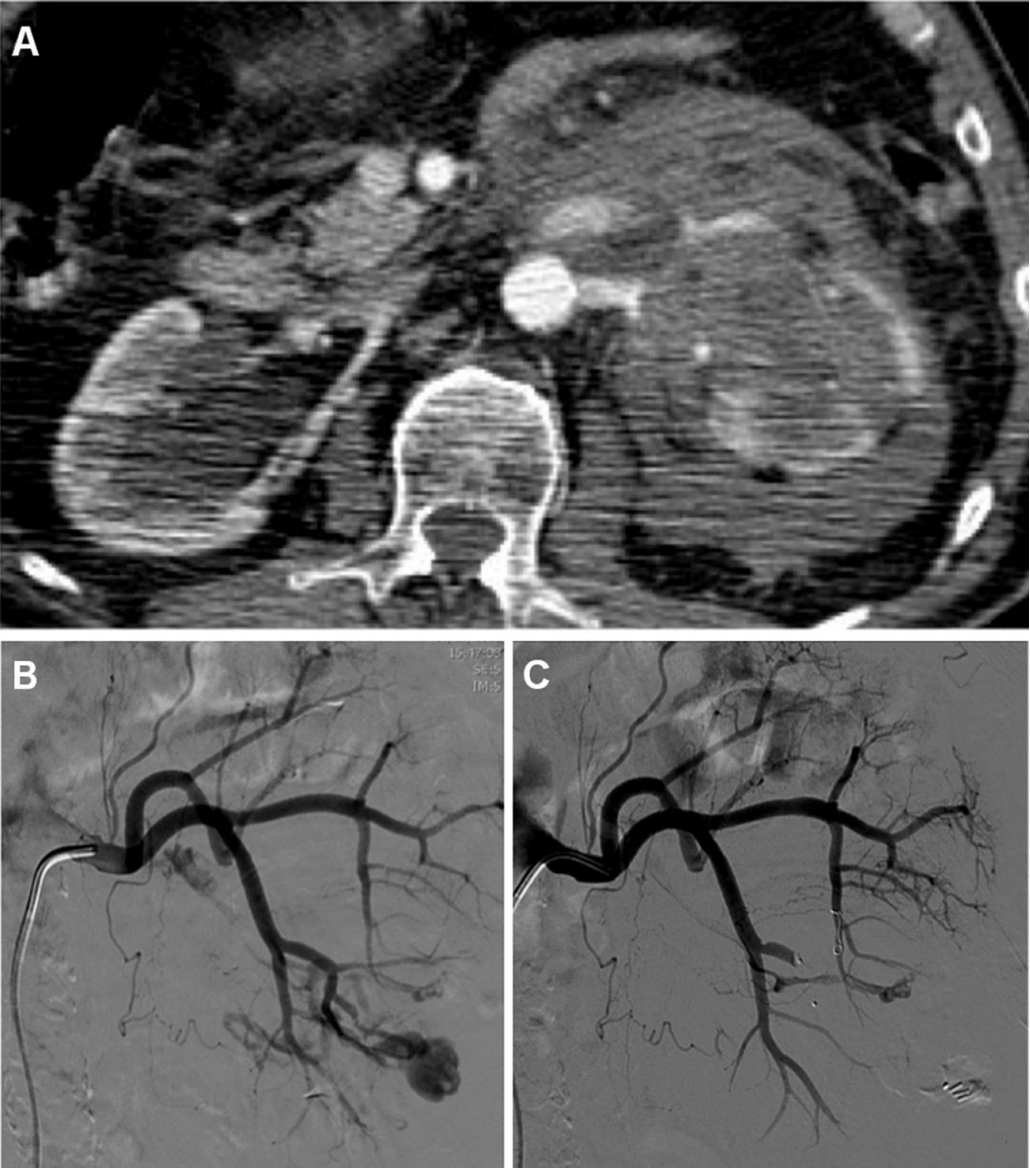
Progressive blood loss, haematuria and ipsilateral flank pain in a 75-year-old man 3 days following enucleation of a left-sided RCC. Arterial-phase CT acquisition (a) revealed a small pseudoaneurysm plus arterio-venous fistula suggested by early opacification of the left renal vein, which were confirmed by urgent angiography (b). After selective embolisation with a 4-mm Amplatzer vascular plug and 2-mm microcoil, repeated angiogram (c) demonstrated complete exclusion of both lesions
